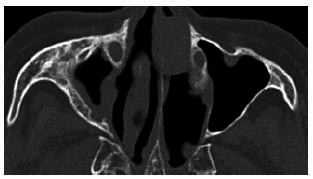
A plain CT scan of the bone window showing noticeable osteolytic bone remodeling in the right maxillary sinus, suggesting the presence of sinusitis.
